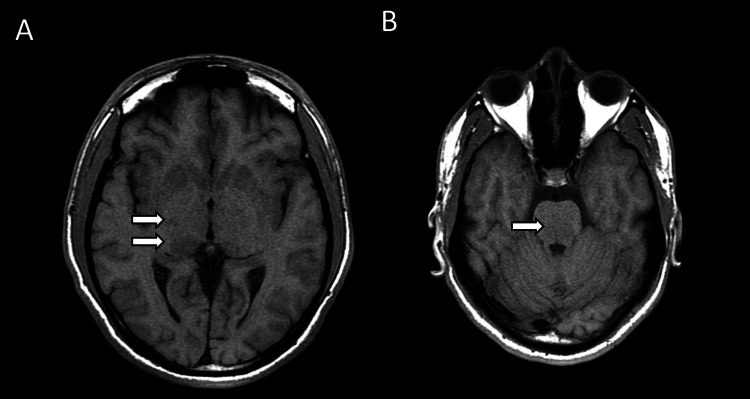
Axial T1-weighted sequence showing multiple hypointense signals in the (A) basal ganglia, thalamus, and (B) brainstem.
